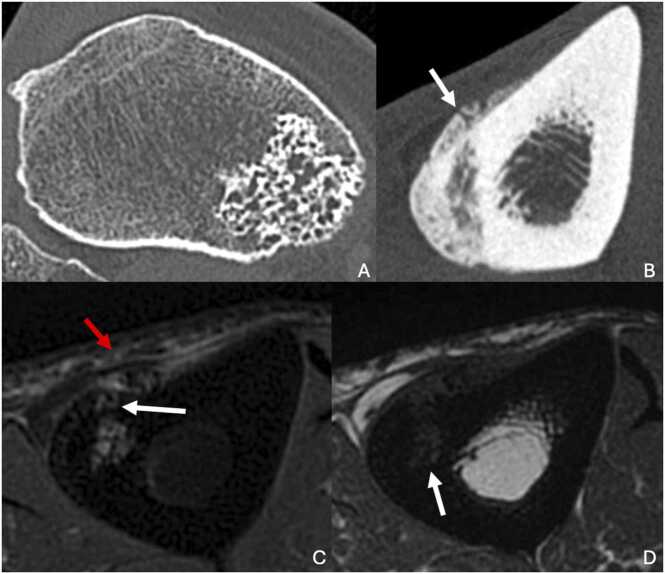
A. Corticomedullar hemangioma of the proximal third of the right tiba in a 53-year-old man presenting medial right knee pain. B,C,D. Cortical hemangioma of the middle third of the left tibia, proven after surgical biopsy, in a 59-year-old man (different patient). A. CT image in the axial plane showing a honeycomb appearance of an eccentric osteomedullar lesion. B. CT image in the axial plane showing cortical thickening with periosteal reaction (white arrow). C. T2-weighted fat-suppressed MR image in the axial plane showing multiple hypersignal aeras separated by hyposignal internal septas (white arrow), with a microvessel inside the cortical thickening (white arrow). Notice the periosteal reaction and soft-tissue adjacent edema (red arrow). D. T1-weighted MR image in the axial plane showing presence of fat in this lesion (white arrow).
